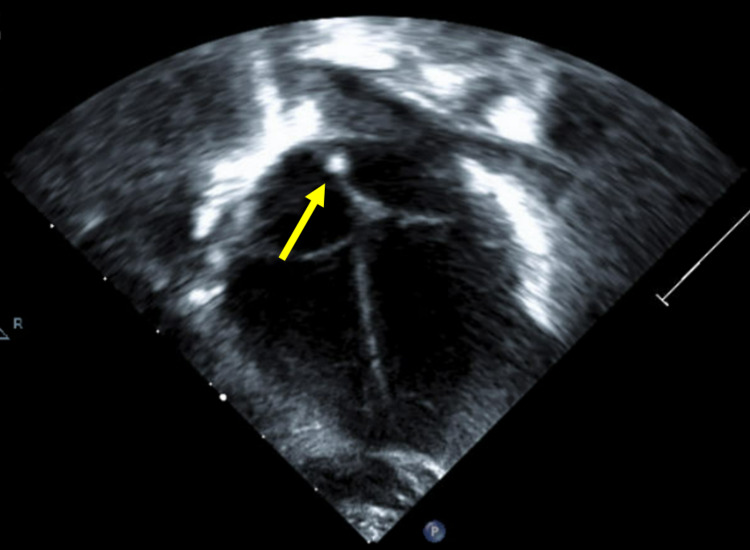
Transthoracic echocardiogram on day 127 with grayscale 4-chamber view demonstrating echogenic foci along the posterior interatrial septum (arrows). No residual curvilinear hyperechoic structure in the hepatic IVC or right atrium IVC: inferior vena cava
